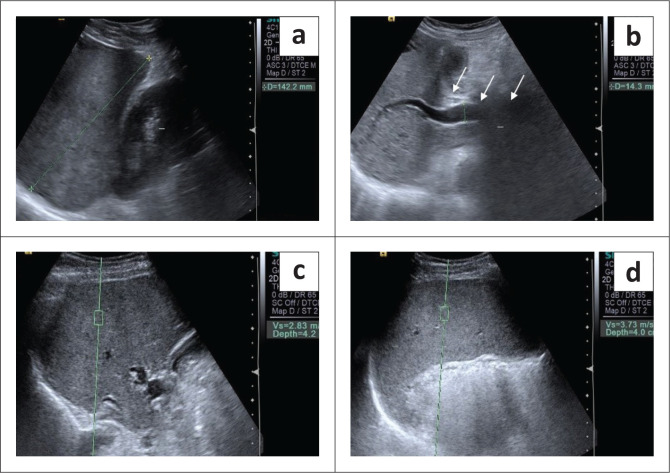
A 39-year-old male with chronic liver disease and oesophageal varices: Grey scale ultrasound reveals a normal sized liver with an altered echo-pattern (a). The portal vein is dilated, measuring 14.3 mm at the porta (b). Hepatic shear wave velocity (SWV) in the liver measures 2.83 m/s (c). Splenic acoustic radiation force imaging measurement shows SWV in this case to be 3.73 m/s (d).
